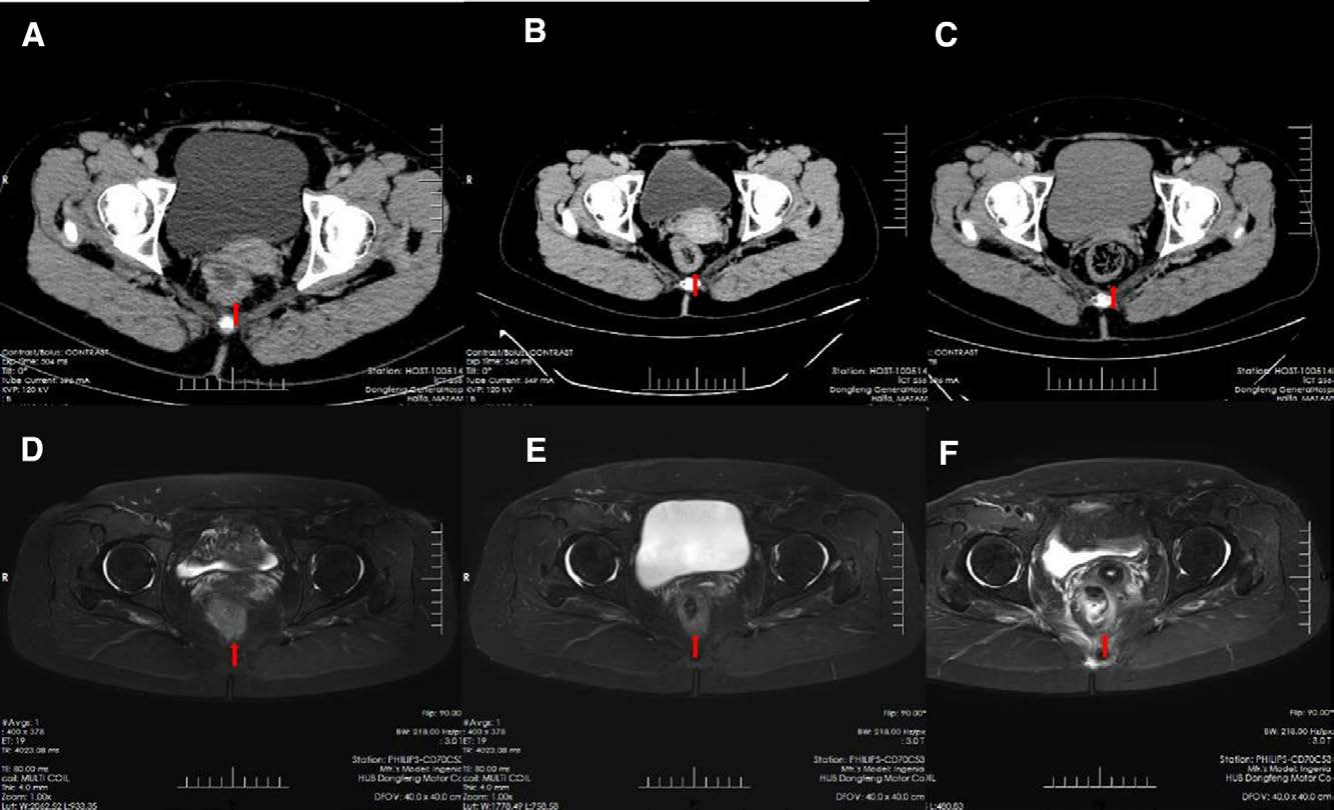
(A) November 2021, enhanced CT showed that the wall of the lower segment of the rectum was unevenly thickened, the mucosa surface was slightly enhanced, and the serous surface was still smooth before treatment. (B) March 2022, after chemoradiotherapy, CT showed that the middle and lower rectal wall was thickened, the mucosa surface was enhanced, and the serous surface was still smooth before cryotherapy. (C) May 2022, after cryotherapy, the middle and lower rectal wall of CT was slightly thickened, the mucosa surface was slightly enhanced, and no obvious tumor was present. (D) November 2021, enhanced MRI sequences of the pelvic cavity showed that the intestinal wall of the middle and lower segments of the rectum was unevenly thickened at a distance of 3.2 cm from the anus, the T2 lipo-pressing sequence showed equal and high signal shadows, the length of which was about 5.0 cm, the serous surface was slightly rough before treatment. (E) March 2022, after chemoradiotherapy, pelvic MR enhanced each sequence showed that the middle and lower segments of the rectum were slightly thickened, the T2 fat pressure sequence showed equal and high signal shadow, the surface of the intestinal wall was smooth, and the enhanced scan of intravenous Magnevist showed slight enhancement before cryotherapy. (F) May 2022, the sequence of enhanced pelvic MR scanning showed that the lower rectal wall was slightly thickened after chemotherapy for rectal cancer, the T2 lipo-pressure sequence showed equal signal shadow, and the surface of the intestinal wall was smooth. CT = computed tomography, MRI = magnetic resonance imaging.
